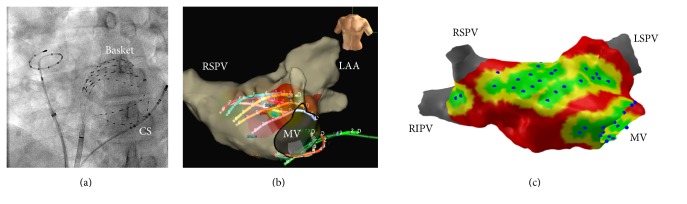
Example for BC deformation. (a) X-ray image of the BC in the LA during the procedure. Note the accumulation of splines at roof and mitral valve annulus. (b) Visualization of the Constellation catheter in the EnSite Velocity system. Eight splines of the basket are visualized in different colors. The CS catheter can be seen in green. (c) The atrial geometry as imported and processed in MATLAB. Blue dots mark the projected surface positions of all electrodes. Areas less than 5 mm away from the electrode surface positions are displayed in green, 5–10 mm in yellow, and >10 mm in red. Areas <10 mm were considered* covered*. All data are from the same patient but do not reflect the same time instance.
